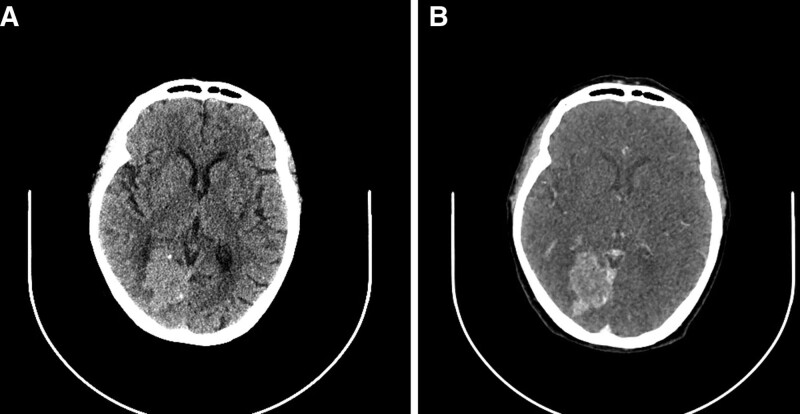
Cranial CT in 2017. (A) An irregular isodense mass in the right occipital region and dotted calcification were found at the edge of the mass. (B) Moderately homogeneous enhancement after contrast materials injected and maximum diameter of the mass is 4.5 cm. CT=computed tomography.
